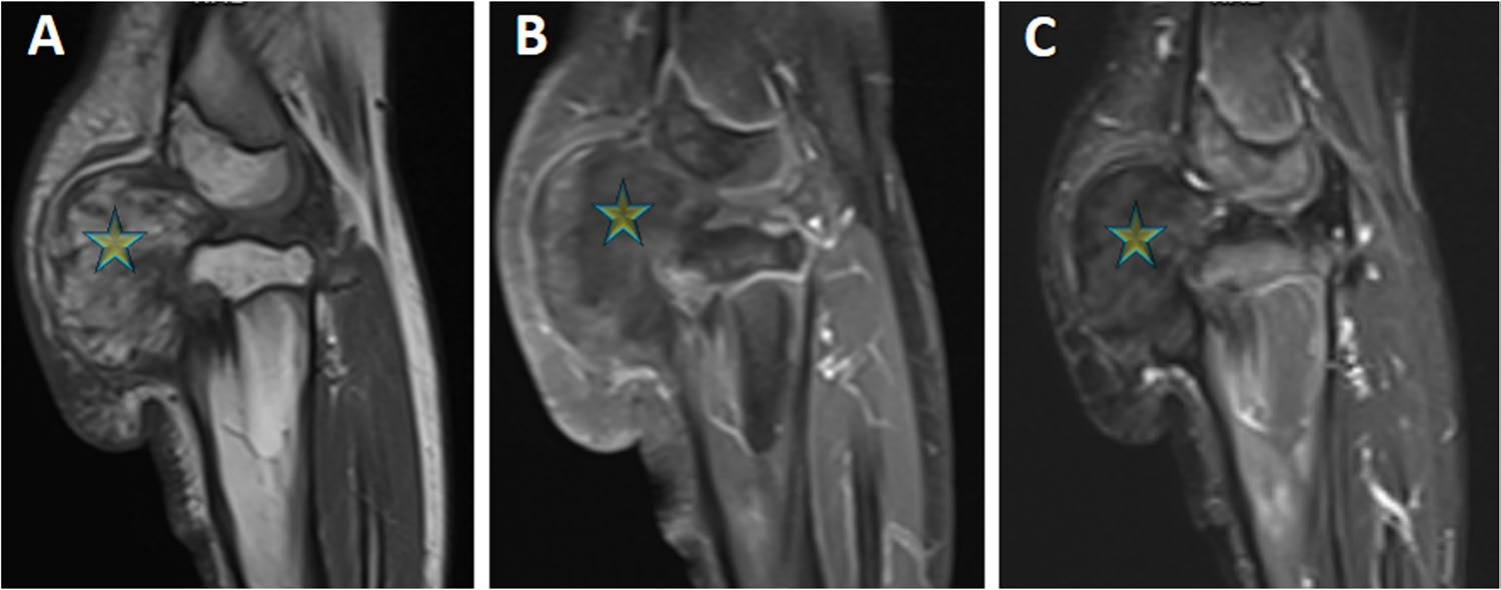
A sagittal non-fat-suppressed T1-weighted MR image (A) reveals a residual or recurrent intra-articular heterogenous-hyperintense lesion (star). Post-contrast fat-suppressed sagittal T1-weighted image (B) shows a minimal contrast enhancement (particularly anteriorly) and signal loss in the fat-containing components. Sagittal fat-suppressed T2-weighted MR image (C) also demonstrates signal loss in the fat-containing components
